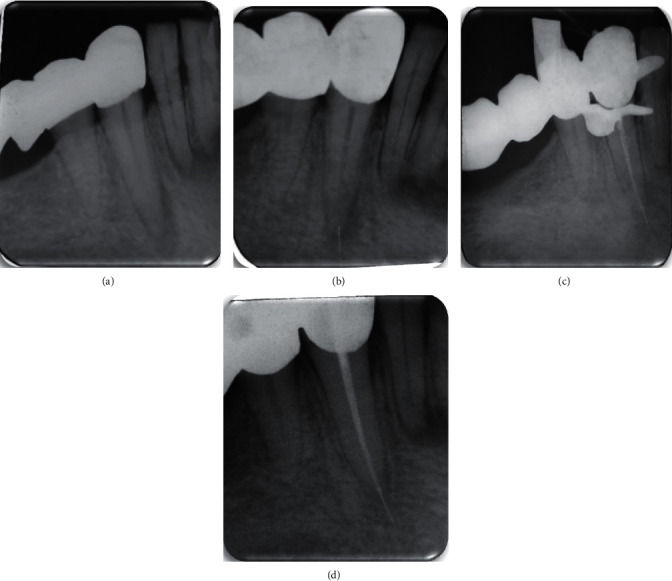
A case of a healed lesion: (a) preoperative radiograph; (b) radiograph showing the fracture of the K20 file; (c) postoperative radiograph; and (d) a 6-month follow-up radiograph.
